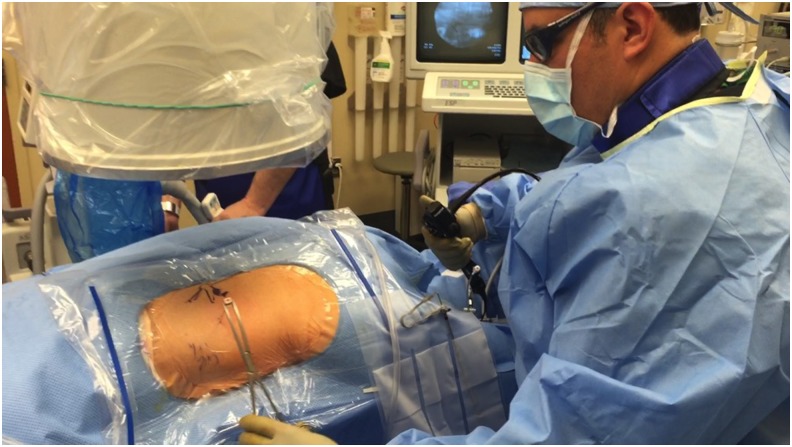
Example of surgeon performing renal access utilizing a combination of ureteroscopic guidance and fluoroscopic radiographic guidance. (Note: photo shown is a left side procedure).
